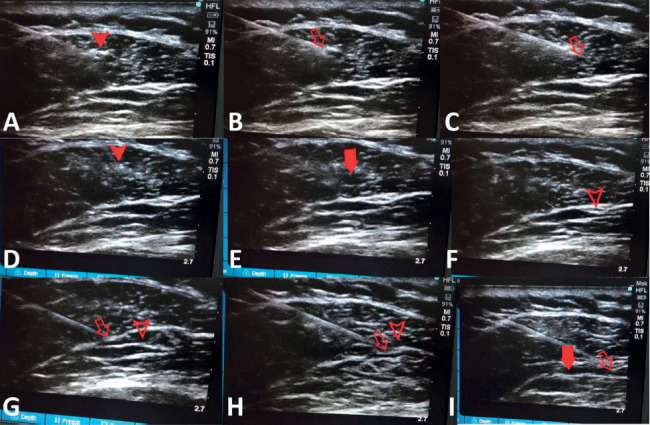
Ultrasound-guided inactivation of trigger points and muscle fascia stripping by liquid knife. The body was examined in advance and marked on the skin with definite tenderness points. The marking points were located in the center of the ultrasonic probe and guided in real time by in-plane technology. (a) Solid triangle refers to the trigger point of the muscle. (b, c) Hollow arrow indicates the tip of the needle, and the trigger point was punctured repeatedly until the patient's aching and distending pain was significantly alleviated and the induced muscle convulsion disappeared. (d) After inactivation of the trigger point, the local echo brightness decreased. (e) Wet needle: liquid of 1 ml was injected into the trigger point, and the solid arrow represents the injected liquid. (f) Hollow triangle refers to muscle fascia. (g) Muscle fascia stretched out by itself when touched by the needle tip. (h) Needle tip pierced into the muscle fascia. (i) Muscle fascia was stripped by the liquid knife, and the solid arrow represents area in the muscle fascia with liquid injected.
